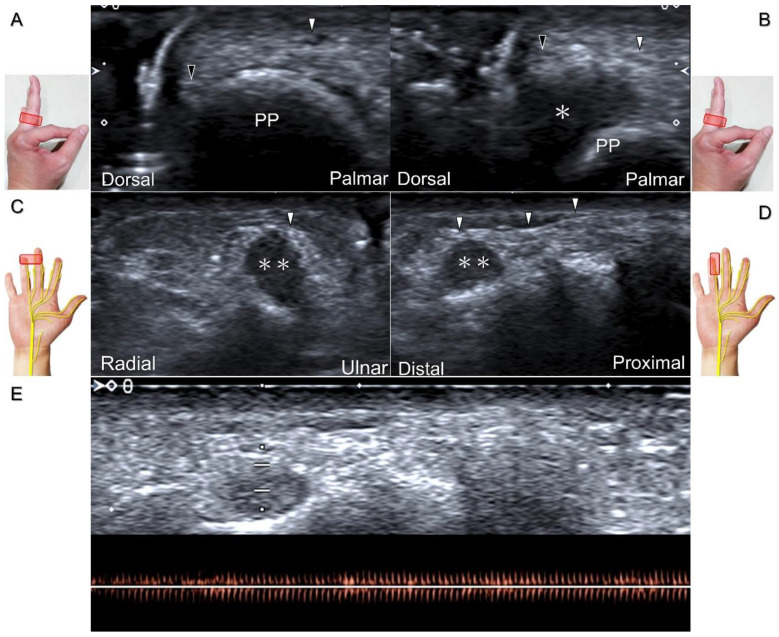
In comparison to the healthy side (A), short-axis imaging (B) shows irritation of both the palmar and dorsal proper digital nerves due to a fibroma (asterisk). Short-axis (C) and long-axis (D) imaging demonstrates irritation of the palmar proper digital nerve due to a hemangioma. Spectral Doppler mode (E) confirms the hemangioma. White arrowhead: palmar proper digital nerve; black arrowhead: dorsal proper digital nerve; double asterisk: hemangioma; PP: proximal phalanx.
